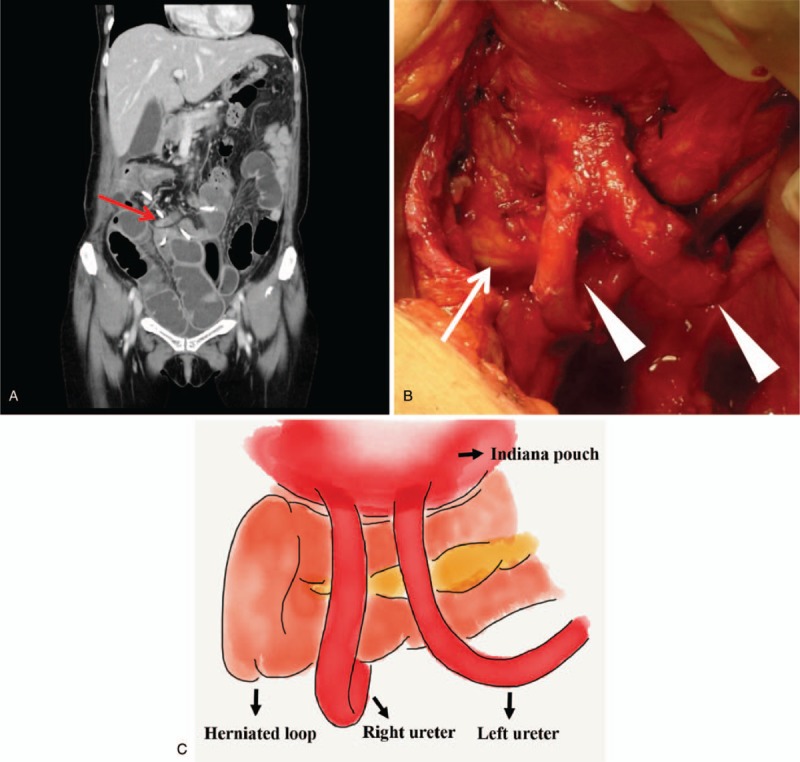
(A) Abdominal CT showed herniated loop between bilateral ureters and caused bowel obstruction (arrow: herniated bowel segment; arrowhead: ureter). (B) Bowel segment herniation and adhesion between bilateral ureters and pouch identified during laparotomy. (C) Sketch of the relative position of the bilateral ureter, urinary diversion, and the hernia loop.
